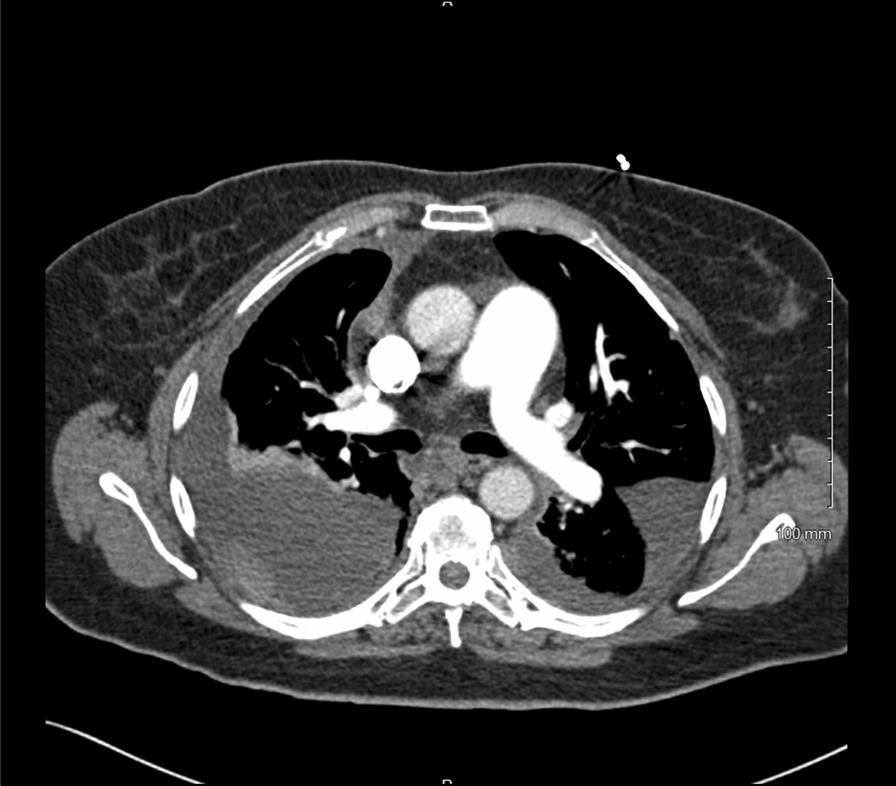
Computed tomography of the chest showing the large bilateral pleural based nodularities
